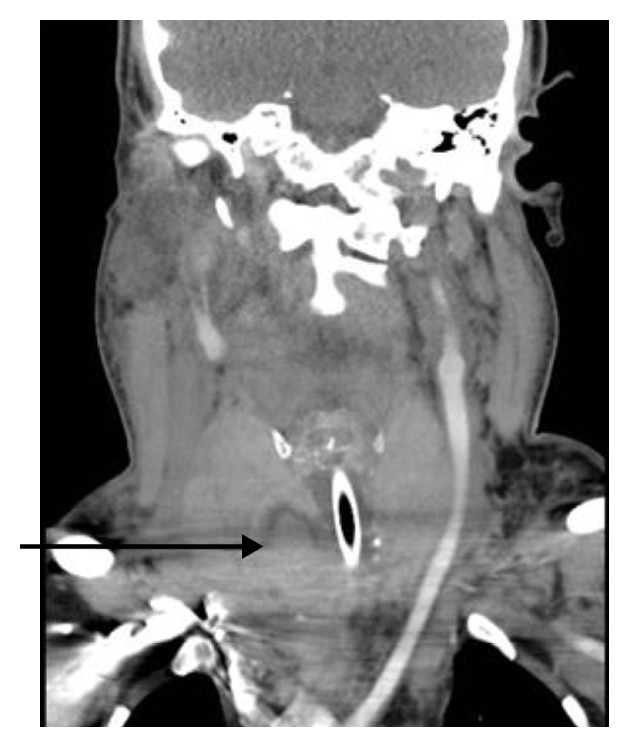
Arterial phase CT neck: coronal view of neck haematoma demonstrating lateral displacement of the great vessels and trachea and hyperdense haemorrhage within right-sided thyroid nodule (arrow).
